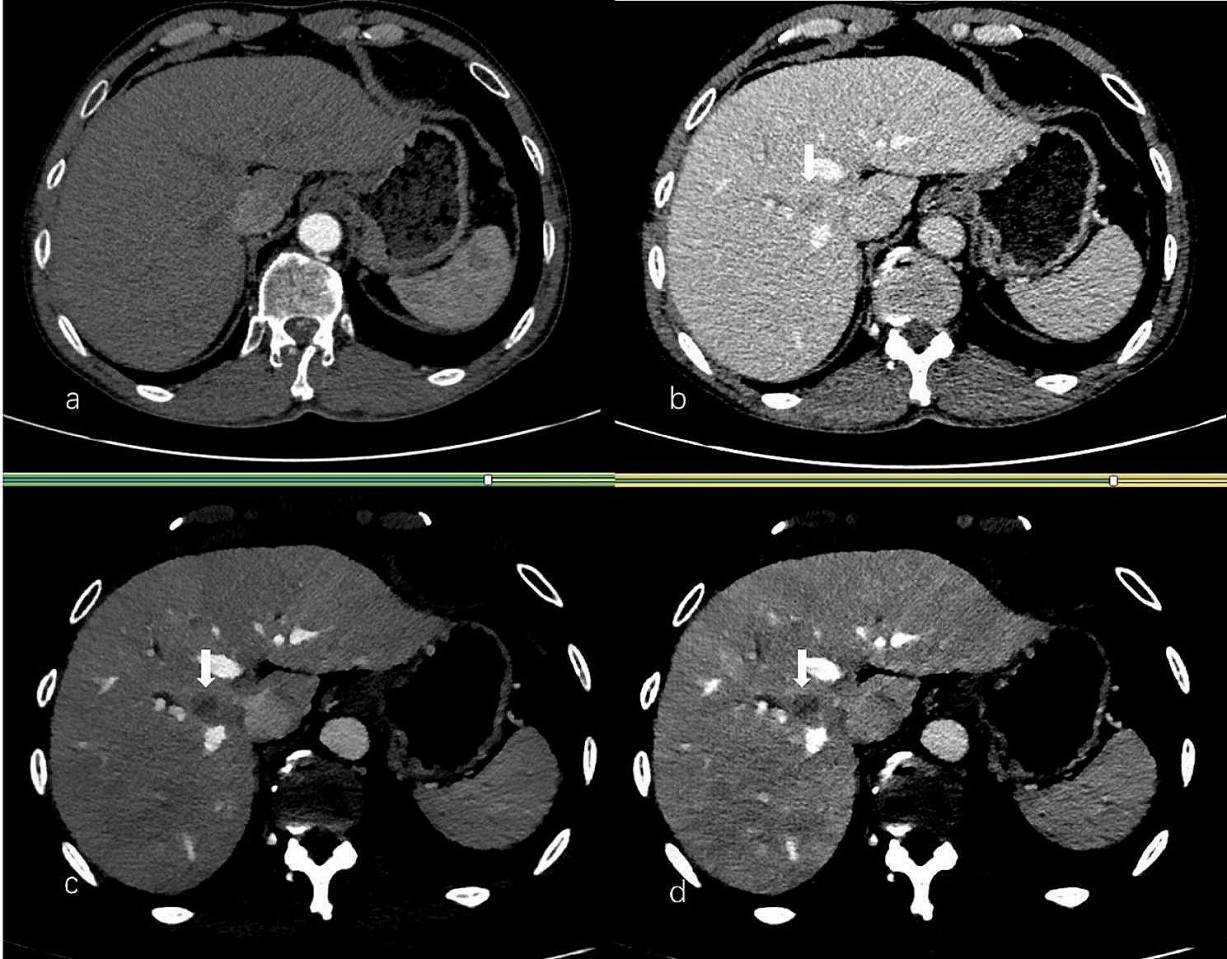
Artery-phase (AP) and portal-vein-phase (PVP) conventional CT image (a, b); PVP virtual monoenergetic images at 40 keV (c); and Gen-VMI_40keV_ (d) produced by the best generative model in a patient with HCC (slice thickness of 3 mm). On the conventional AP contrast-enhanced CT images, no lesion was visible (a). The lesion was faint in PVP (arrow, b). PVP virtual monoenergetic images at 40 keV and Gen-VMI_40keV_ showed HCC, which are more conspicuous (arrow, c&d)
